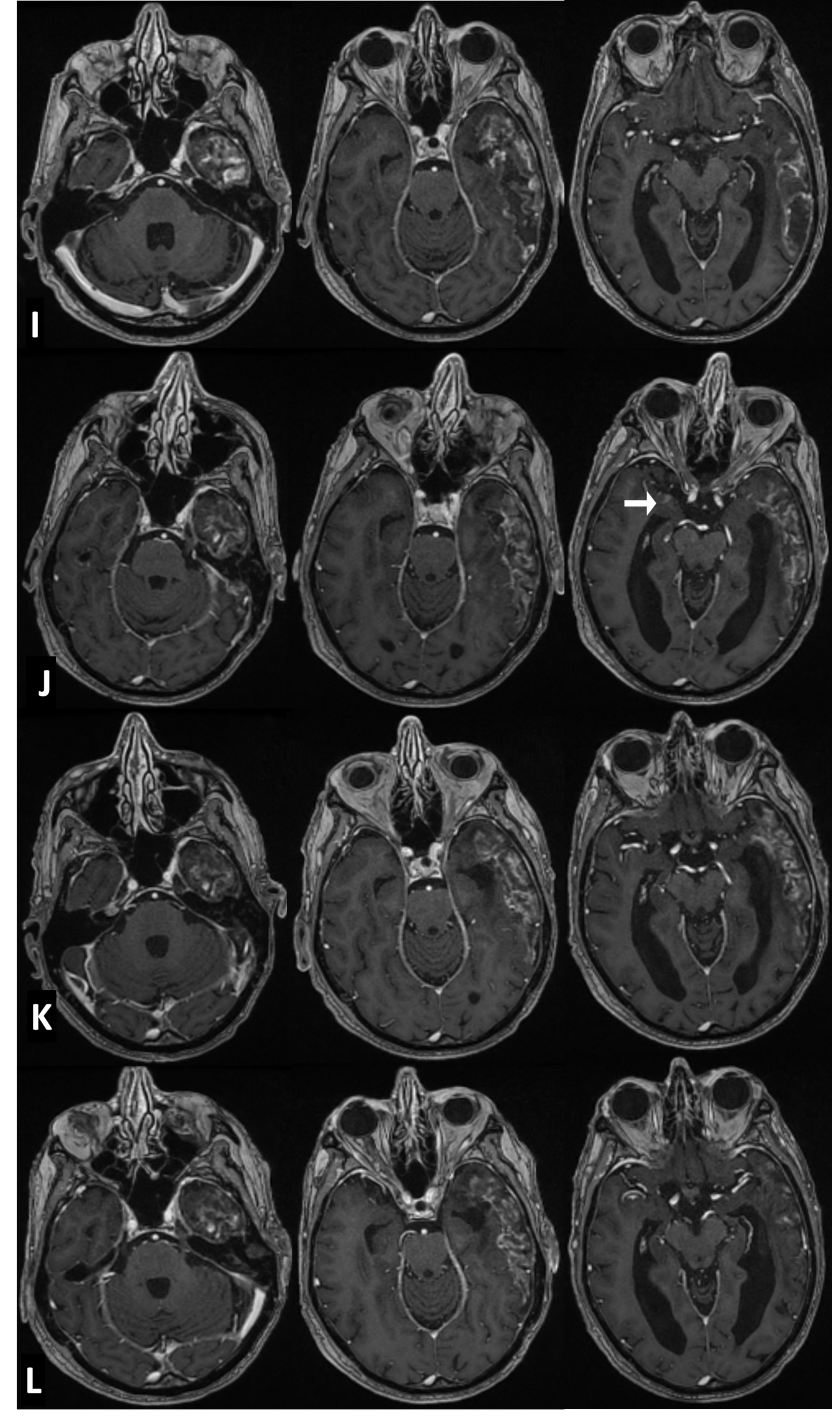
Follow-up axial post-contrast MRI images I- At three months, no change in enhancement. J- At nine months, development of new contralateral temporal lobe enhancing lesion (white arrow). K- At 18 months, mild temporal lobe atrophy with stable enhancement pattern in both the lesions. L- At 24 months encephalomalacia with ex-vacuo dilatation of the temporal horn and marked temporal lobe atrophy, resolution of contralateral enhancing lesion.
